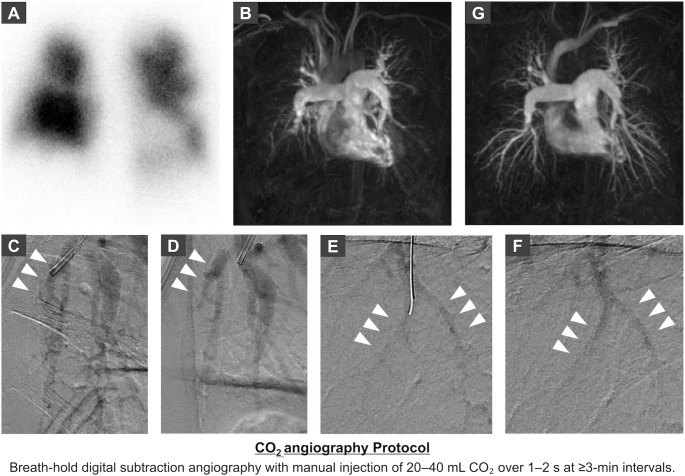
A Ventilation–perfusion scintigraphy. B, G Contrast-enhanced MRA (pre- and post-BPA). C–F CO₂ angiography of Lt.A10 (pre- and post-BPA) and Rt.A10 (pre- and post-BPA)
